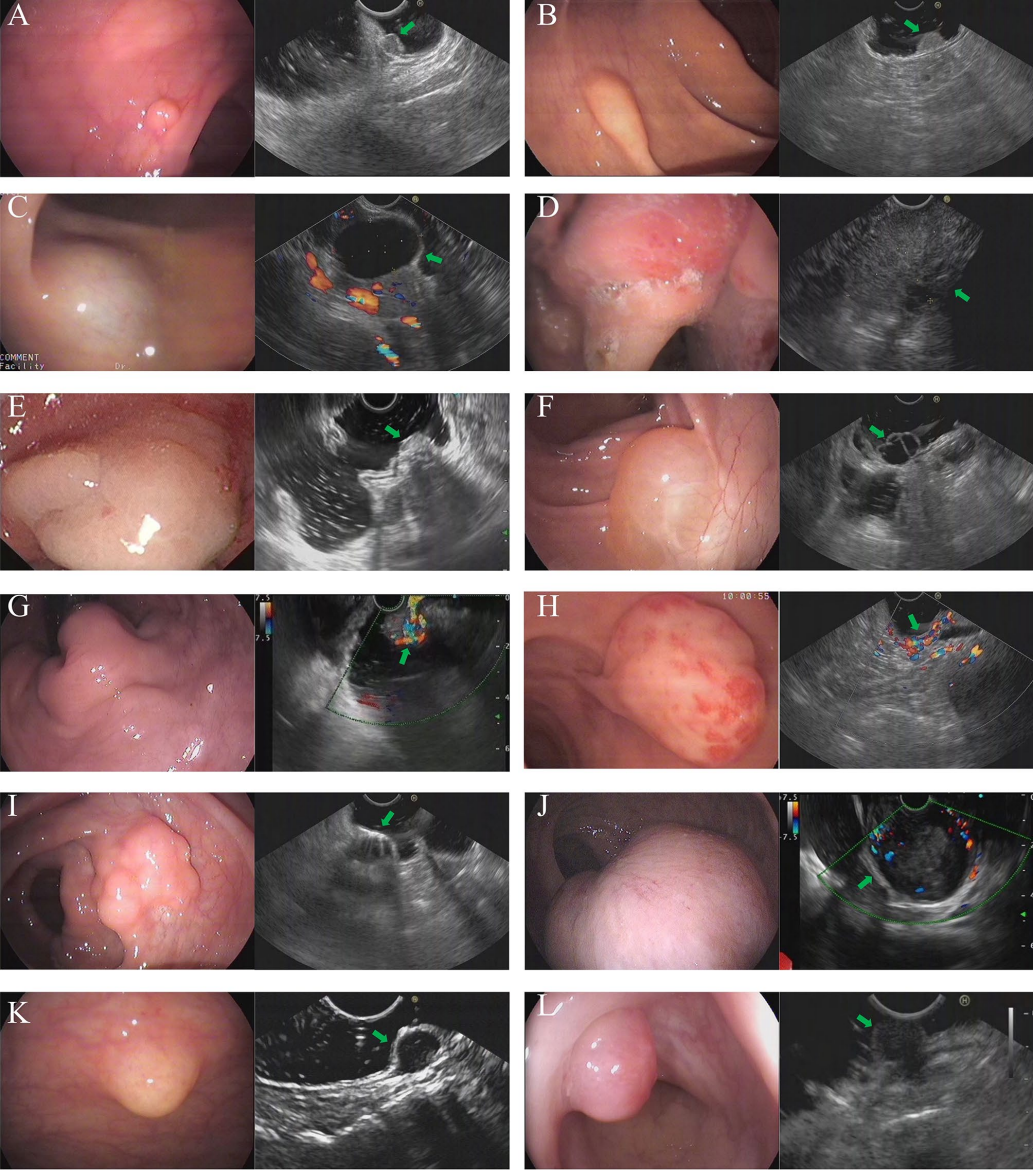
Endoscopic and ultrasonographic images of lower gastrointestinal subepithelial lesions. (A) Neuroendocrine tumor (NET); (B) Lipoma; (C) Renal cyst extraluminal compression; (D) Cancer; (E) Inflammation; (F): Cyst; (G) Varix; (H): Glomus; (I) Pneumatosis cystoides intestinalis (PCI); (J) Gastrointestinal stromal tumor (GIST); (K) Schwannoma; (L) Endometriosis.
